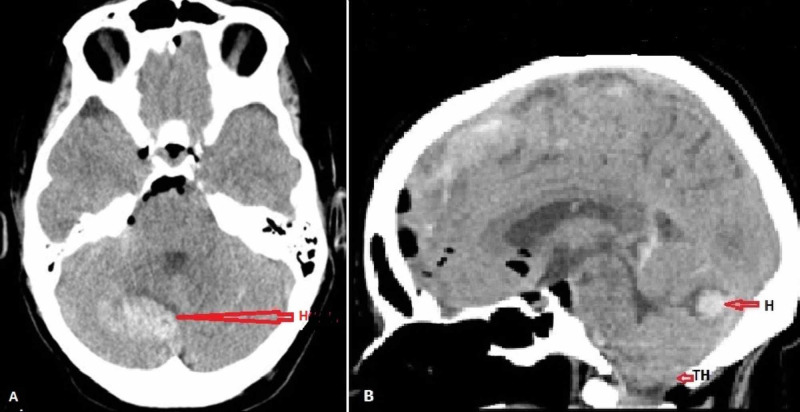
(A) CT axial section showing cerebellar hemorrhage (H). (B) CT sagittal section showing cerebellar hemorrhage (H) and tonsillar herniation (TH).
